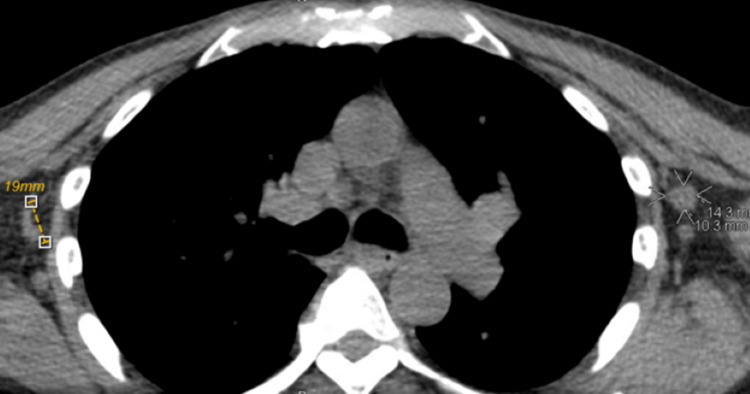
CT chest CT: computed tomography
